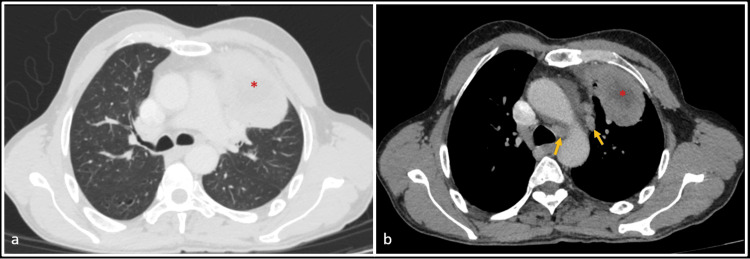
CT scan images of the patient's lungs (axial view) Axial contrast-enhanced computed tomography (CT) scans with lung (a) and soft tissue windows (b) show a large heterogeneously enhancing mass in the left upper lobe (red asterisk). Enlarged suspicious para-aortic and left lower paratracheal nodes are also seen (yellow arrow).
